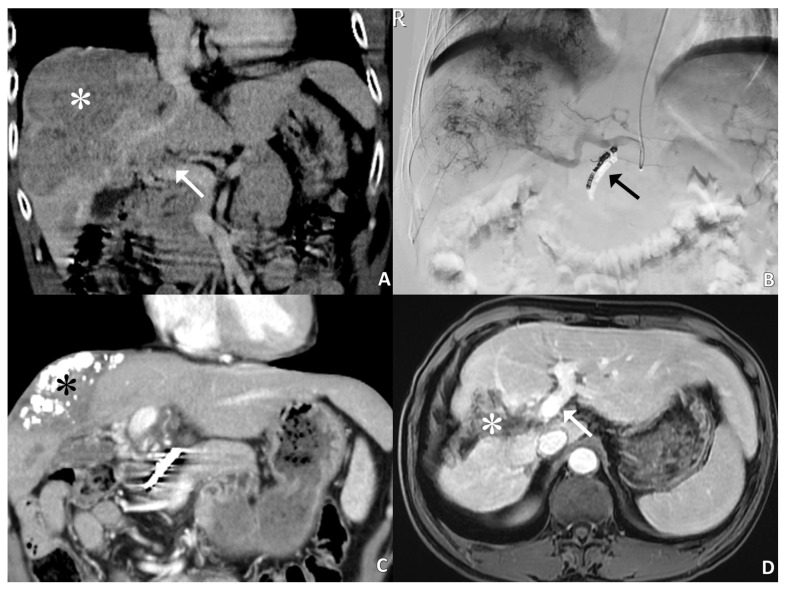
A 55-year-old male patient with complete response after five cycles of HAIC. (A): Coronal CT image shows a large HCC (white asterisk) in the right lobe of the liver with main portal venous tumor thrombosis (arrow). The initial serum AFP level is 35,678 ng/mL. (B): Hepatic angiogram reveals large tumor burden. The gastroduodenal artery is embolized with microcoils (arrow). (C): Partial response is obtained after two cycles of HAIC treatment. The hyperattenuated region (black asterisk) within the tumor is lipiodol retention. (D): Follow-up MRI after 5 cycles of HAIC treatment shows tumor shrinkage with cystic necrosis and lipiodol retention (white asterisk) in addition to complete resolution of portal venous tumor thrombosis (arrow). The serum AFP level is 2.5 ng/mL, and the patient has survived for more than 3 years after initial diagnosis of advanced HCC with PVTT.
